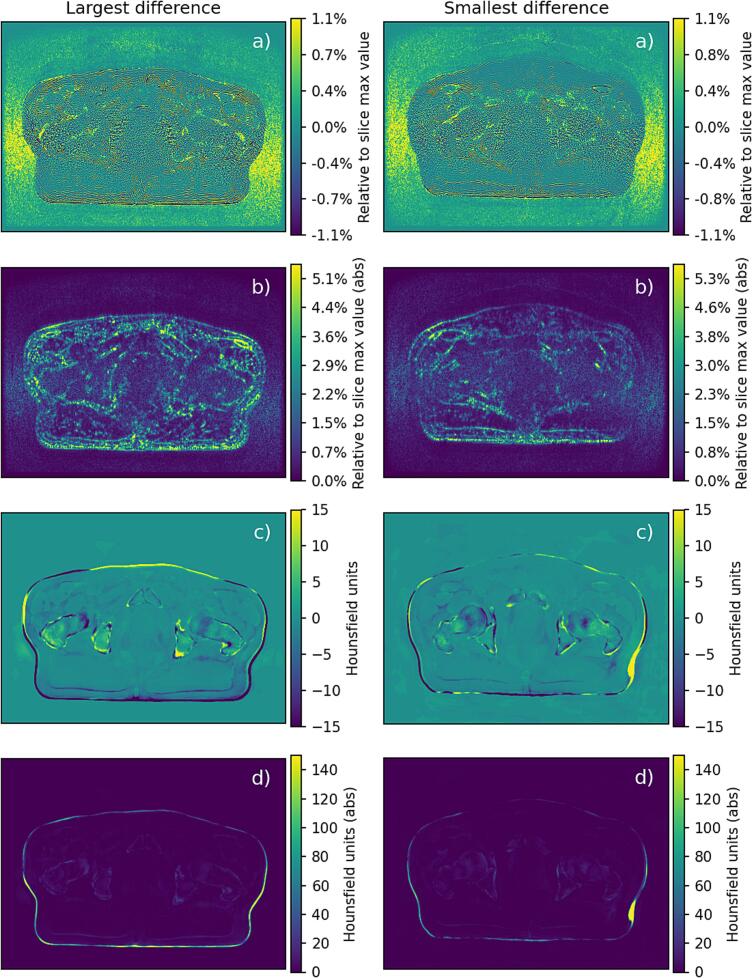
The figures represent differences between MRI using conventional reconstruction and DLR and their corresponding sCT in cohort 1. The left-hand side originates from the patient with the largest absolute value of body volume difference (27.2 cm^3^), and the right-hand side originates from the patient with the smallest absolute value of body volume difference (3.5 cm^3^). The images represent the following: a) Difference image between MR volumes with conventional and DLR reconstruction. Notice the homogeneous content of noise and Gibbs ringing artefacts. Also notice that the image differences do not geometrically correspond to the image differences seen for the sCT. b) Absolute value of image in a) with a wider display window for improved visualization. c) Difference image of the two corresponding sCT volumes for a window width of −15 to 15 Hounsfield units (HU). d) Absolute value of image in c) with a wider window of 0 to 150 HU for improved visualization of peripheral differences. The different window width and level enhance visibility, with higher signal values than the defined scale adopting the color of the highest defined window value. Notice the residual difference in left and right side of the patient for the patient with the largest difference and the small deviation on the patient left side for the patient with the smallest absolute body volume difference (HU scale saturated, true difference value was larger than 150).
